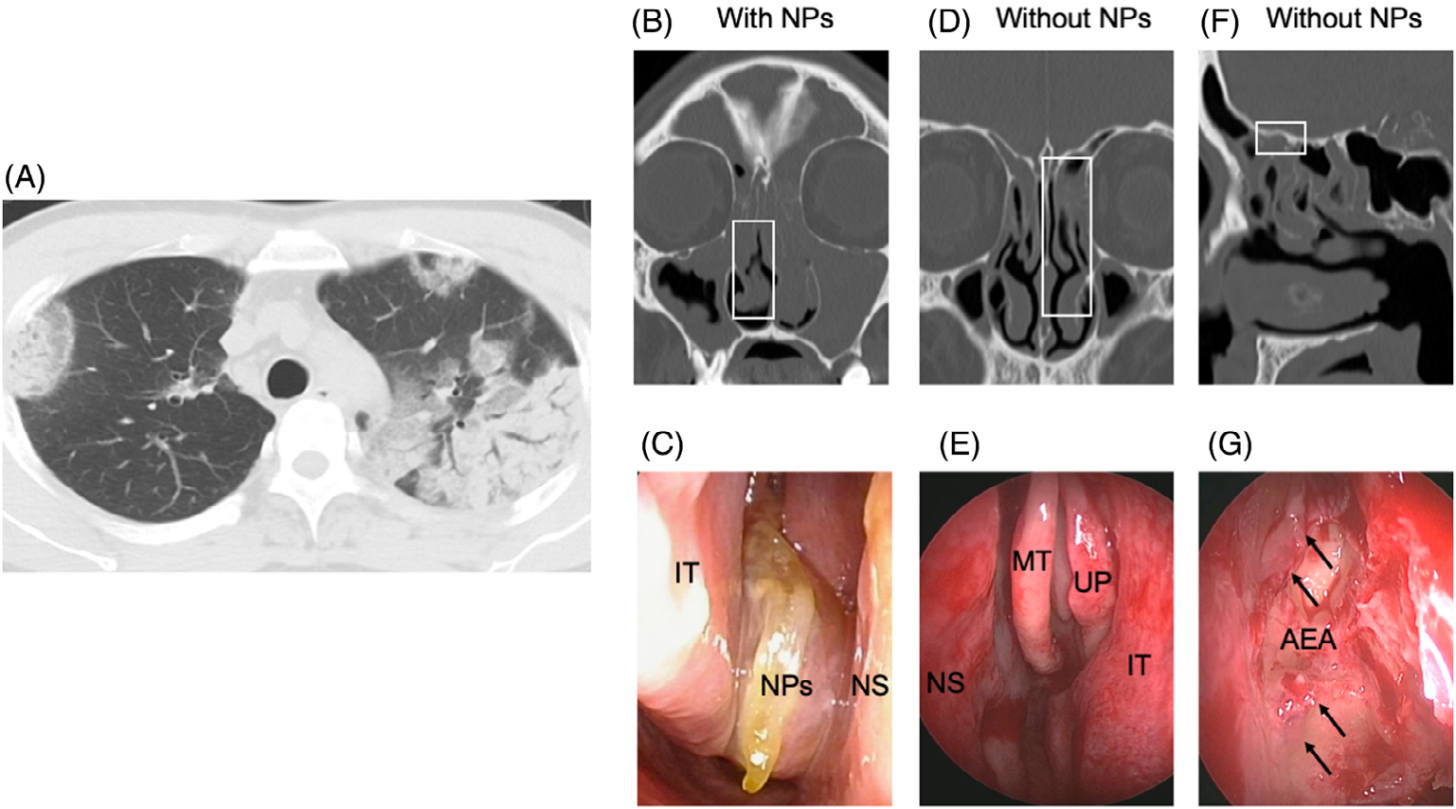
Representative imaging findings of comorbid CRS at onset of CEP. Representative chest CT findings in ID 1 (A). Representative findings of comorbid CRS with (B, C) and without NPs (D–G) in CEP: Sinonasal CT findings (B, D, and F); Endoscopic findings (C, E, and G). The figures B and C were in ID 1, while figures D, E, F, and G in ID 5. Black arrows indicate the edematous mucosa in the ethmoidal tissues. Endoscopic findings correspond with the squares in the CT findings. AEA, anterior ethmoidal artery; CEP, chronic eosinophilic pneumonia; CRS, chronic rhinosinusitis; CT, computed tomography; IT, inferior turbinate; MT, middle turbinate; NPs, nasal polyps; NS, nasal septum; UP, uncinate process.
